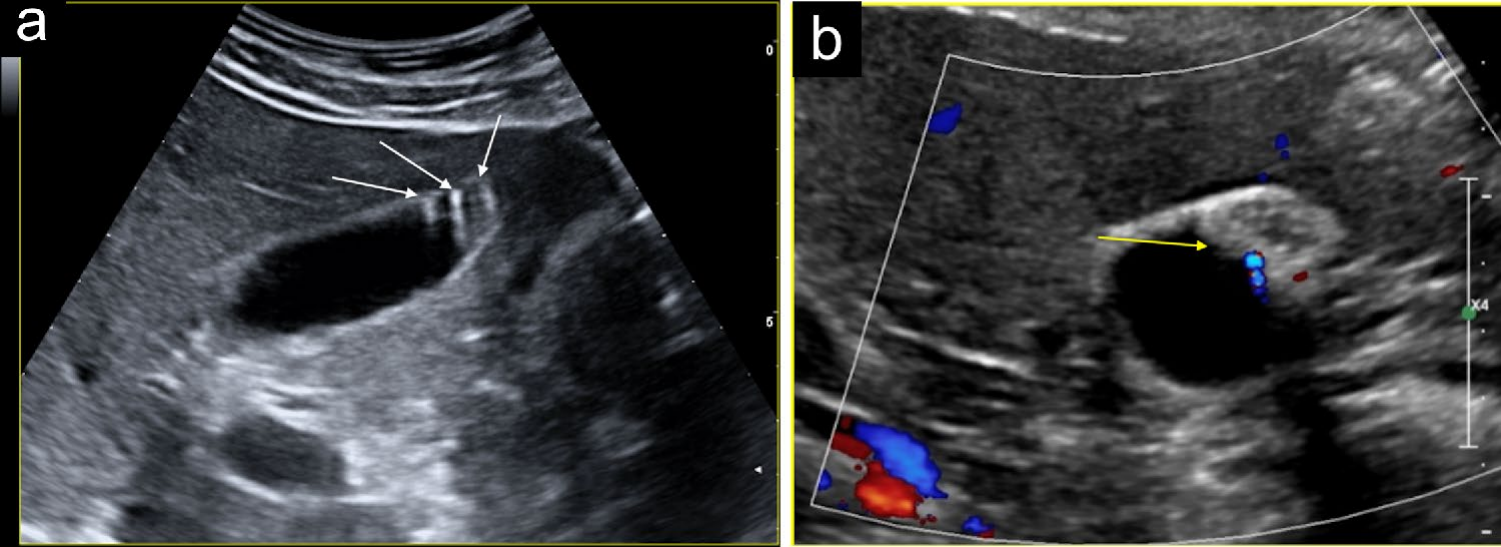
a The sonographic images show the localised type of GA as a focal thickening localised in the fundus. The thickening projects into the lumen and appears hypoanechoic with hyperechoic cholesterol crystals generating comet-tail reverberation artifacts (white arrows). b The sonographic images show the localised type of GA as a focal thickening localised in the fundus. The thickening projects into the lumen and appears hypoanechoic with hyperechoic cholesterol crystals generating twinkling artifacts on the colour Doppler exam (yellow arrow)
